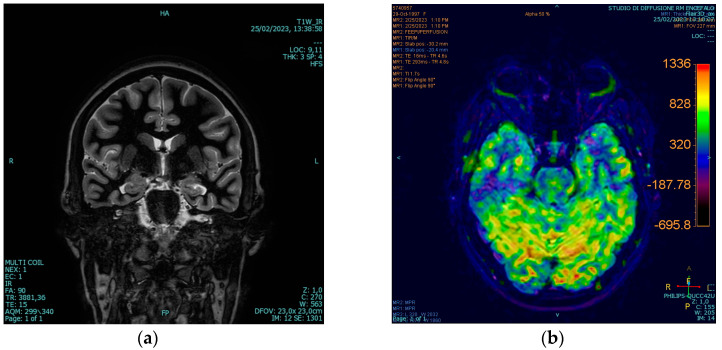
Three-Tesla MRI images and diffusion-weighted imaging (DWI) acquired. (a) Dysplastic lesion in right amygdala; (b) perfusion-weighted sequences.
